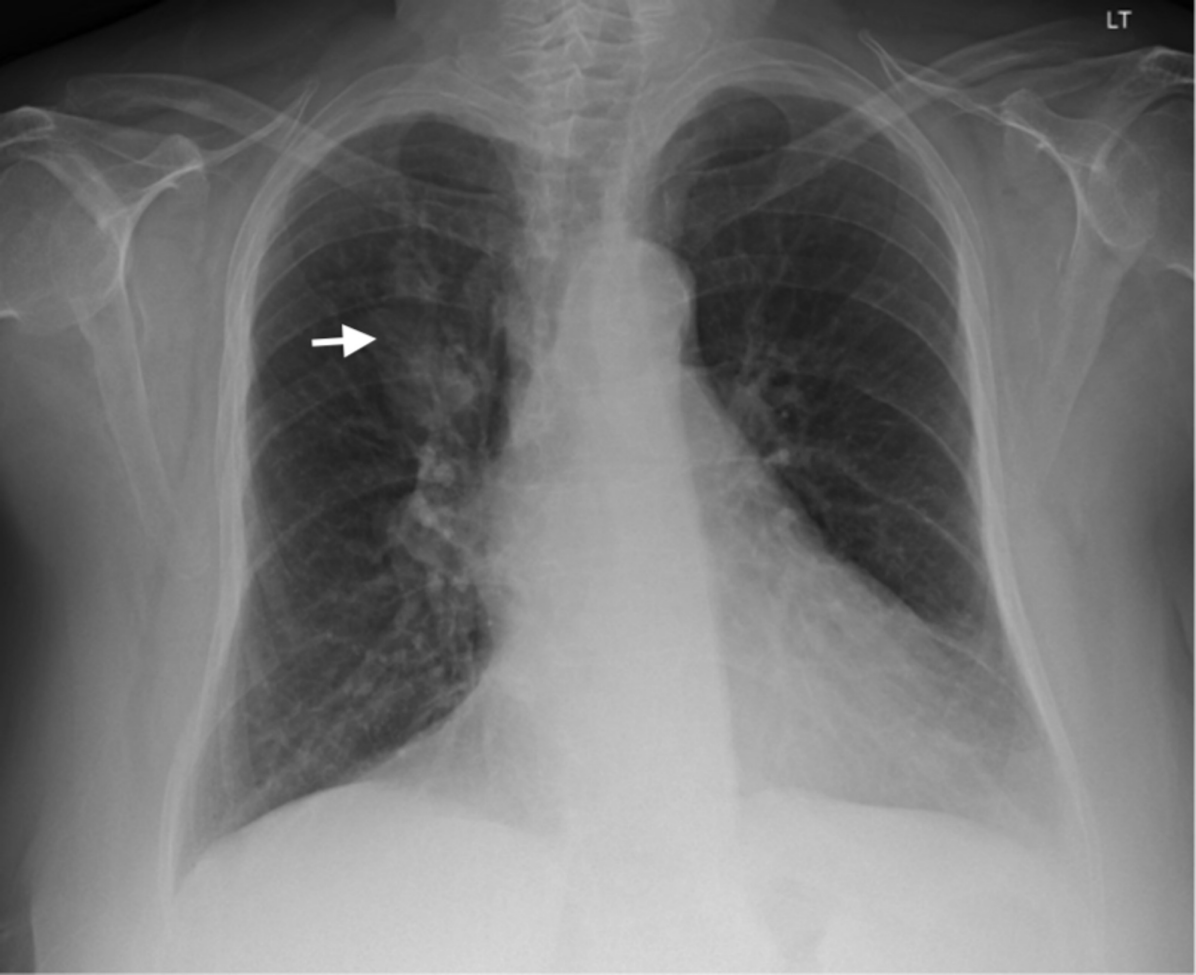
Chest Xray The image demonstrates the presence of a right upper lobe opacity (arrow).
